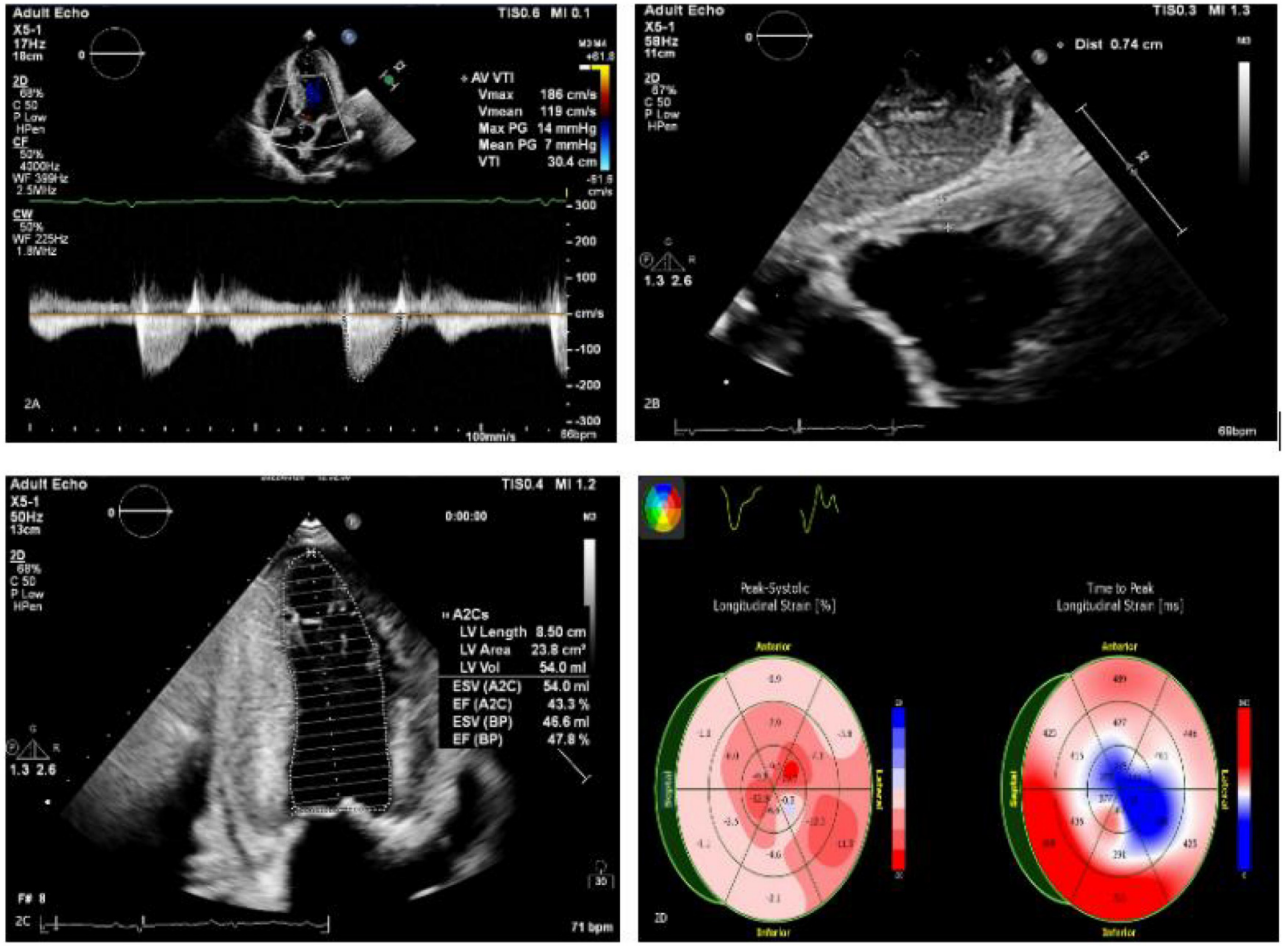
(A–C) Echocardiography findings include an enlarged left atrium and symmetrical thickening of the left ventricular myocardium (LVPWd 1.48 cm). Dense, granular sparkling echoes in the myocardium are observed, along with thickened right ventricular walls (free wall thickness 0.74 cm). Both mitral and tricuspid valves are thickened with mild regurgitation. There is reduced left ventricular systolic function (EF: 47.8%) and severely reduced left ventricular diastolic function (grade 3). A small amount of pericardial effusion is present. (D) The left ventricle shows preserved apical strain, presenting as a “cherry on top” sign. The left ventricular global longitudinal strain (LV GLS) is −6.2% (Left Ventricular Global Longitudinal Strain is a sensitive parameter for assessing left ventricular myocardial systolic function, derived from speckle-tracking echocardiography).
